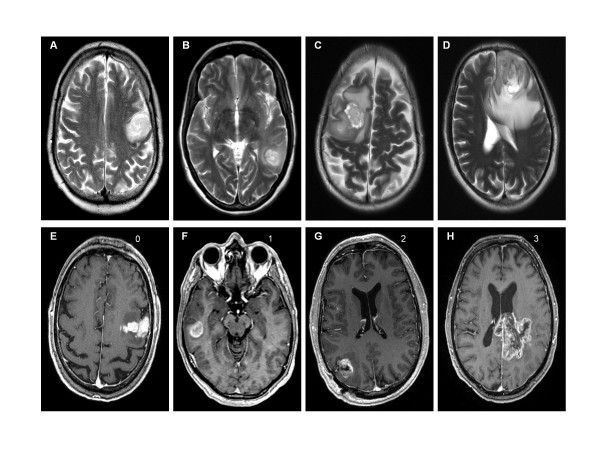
Examples for two-dimensional measurement and scoring of edema (A-D) and necrosis (E-H). A-D, scoring of edema (t2-w -contrast MRI). E-H, scoring of degree of necrosis (t1-w +contrast MRI).
